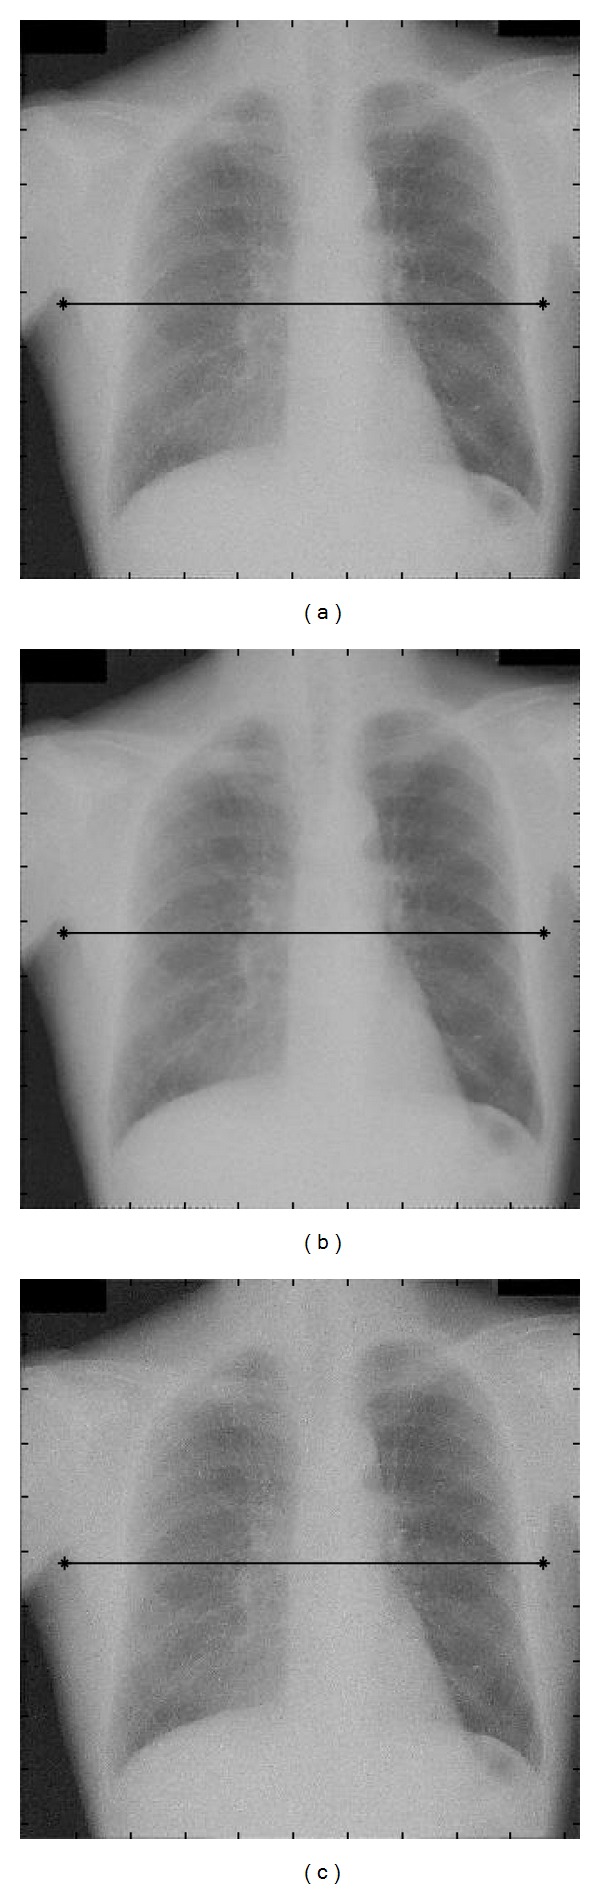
Image processing results for chest radiographs. (a) Image processed by the proposed method, (b) image processed by the modified UDWT method, and (c) image processed by the sigmoid-type wavelet coefficient mapping method.
